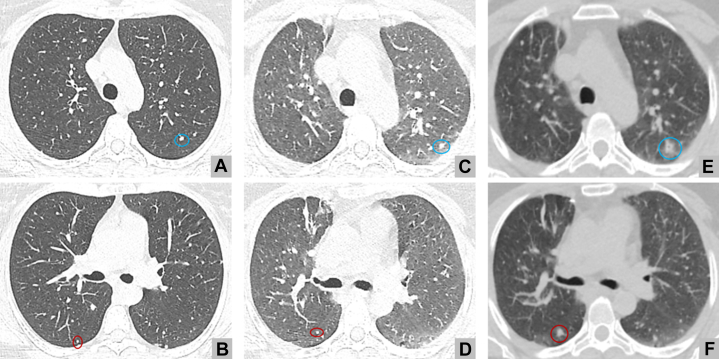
A-F, Serial chest CT imaging demonstrating interval growth of pulmonary nodules. A-B, Initial CT scan (corresponding to Fig 1). C-D, Follow-up scan 2 y later. E-F, Most recent CT scan, approximately 1 y after the previous imaging. The left upper lobe nodule, marked in a blue circle, and the right lower lobe nodule, marked in a crimson circle, both demonstrate interval growth. Notably, the nodule in the left upper lobe (panel E) exhibits central lucency, suggestive of necrosis.
